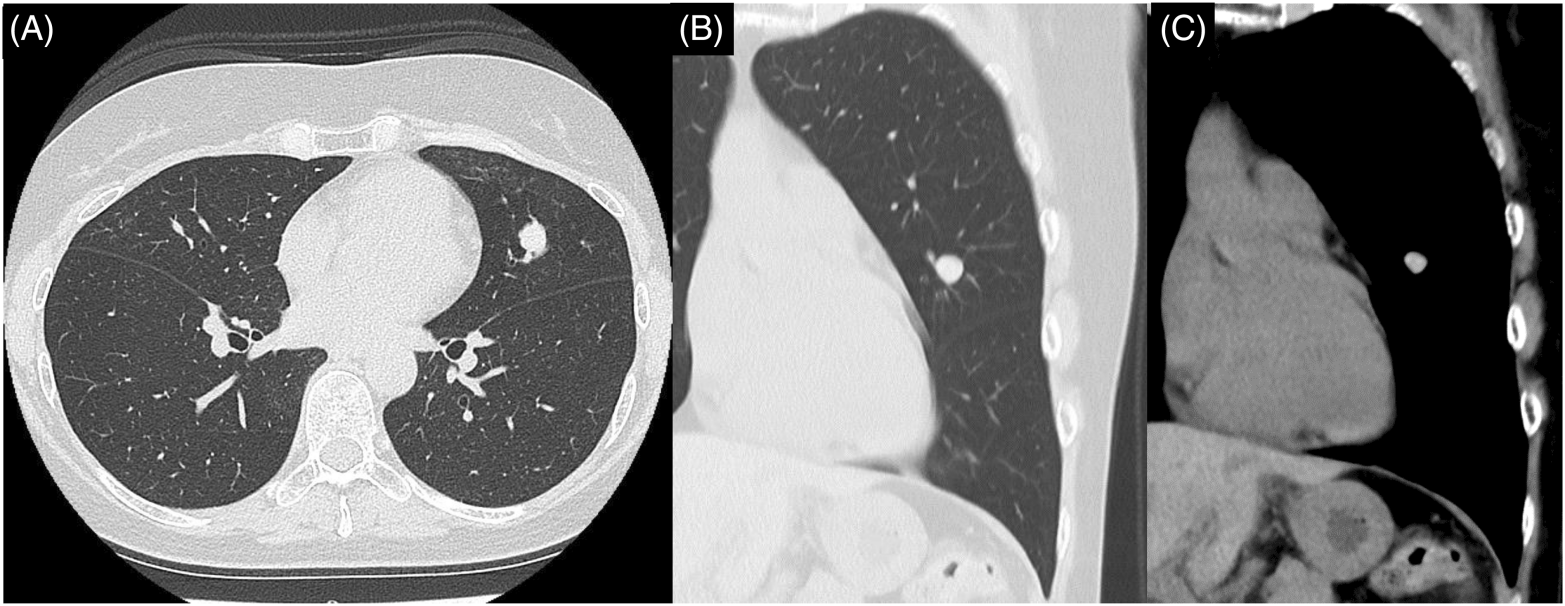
Chest computed tomography in a 53‐year‐old woman with asthma revealed a solid nodule (84 HU) with a maximum diameter of 11 mm in the S5 of the left lung. (A: axial, B: coronal lung window and C: mediastinal window).
